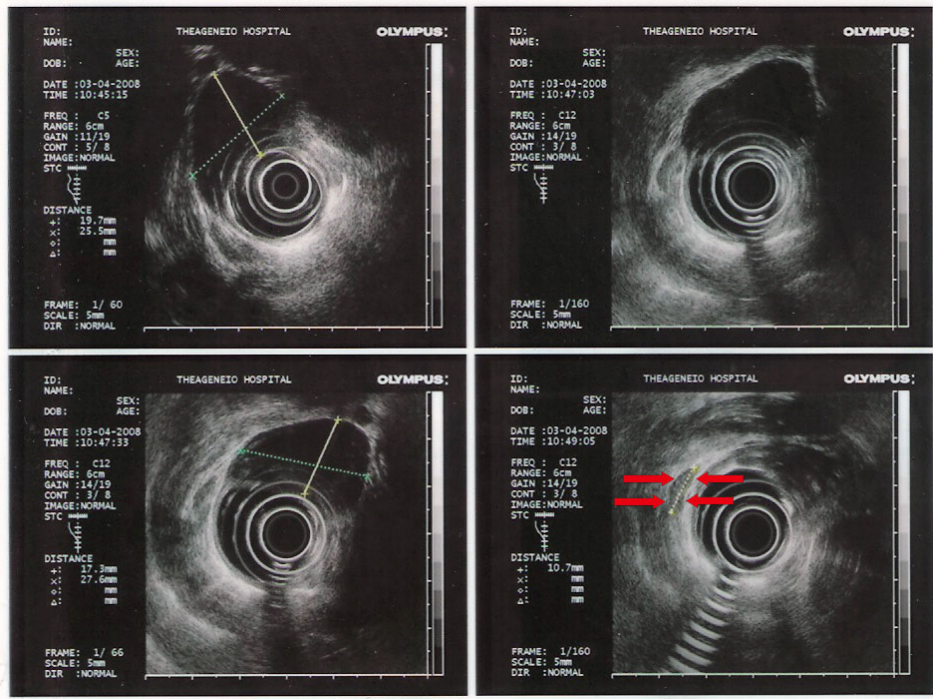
Endoscopic ultrasonographic evaluation of a leiomyoma. The tumor is presented as a well-demarkated, homogeneous and hypoechoic lesion with clear margin, originating from muscularis mucosa. In this case, its size is 2.7 × 1.7 cm. A small lymph node (1.07 cm) is also discovered (red arrows).
